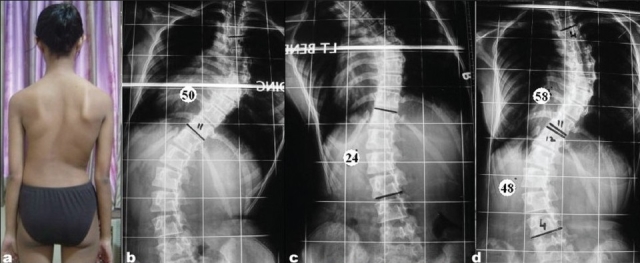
(a) Preoperative clinical picture (a) and whole spine standing anteroposterior (b), right and left bending X-rays (c,d) of typical King type II curve. Thoracic curve (T4-T11) is more and less flexible than lumbar with Cobb's angle 58° in thoracic and 48° in Lumbar
